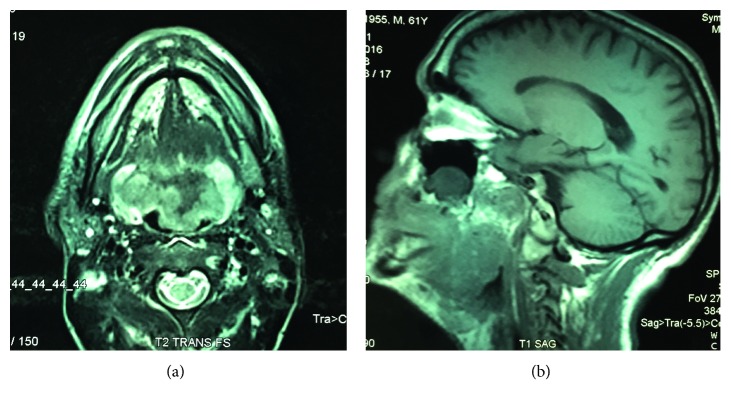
Sagittal and transversal MRI sections showing a voluminous process of the posterior tongue infiltrating the uvula and tonsils.
